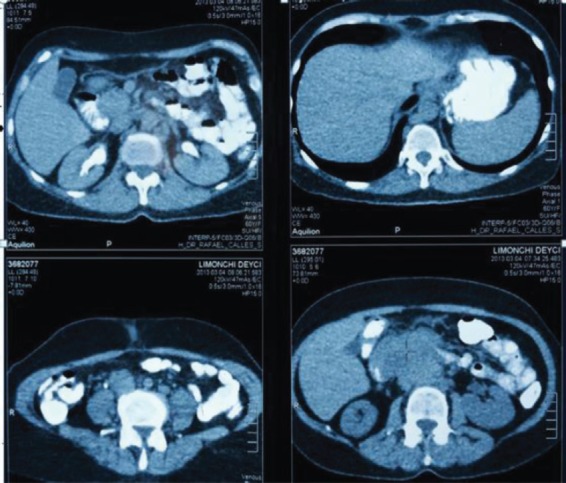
Abdominopelvic CT with oral and intravenous contrast during the elimination phase, axial sections.
